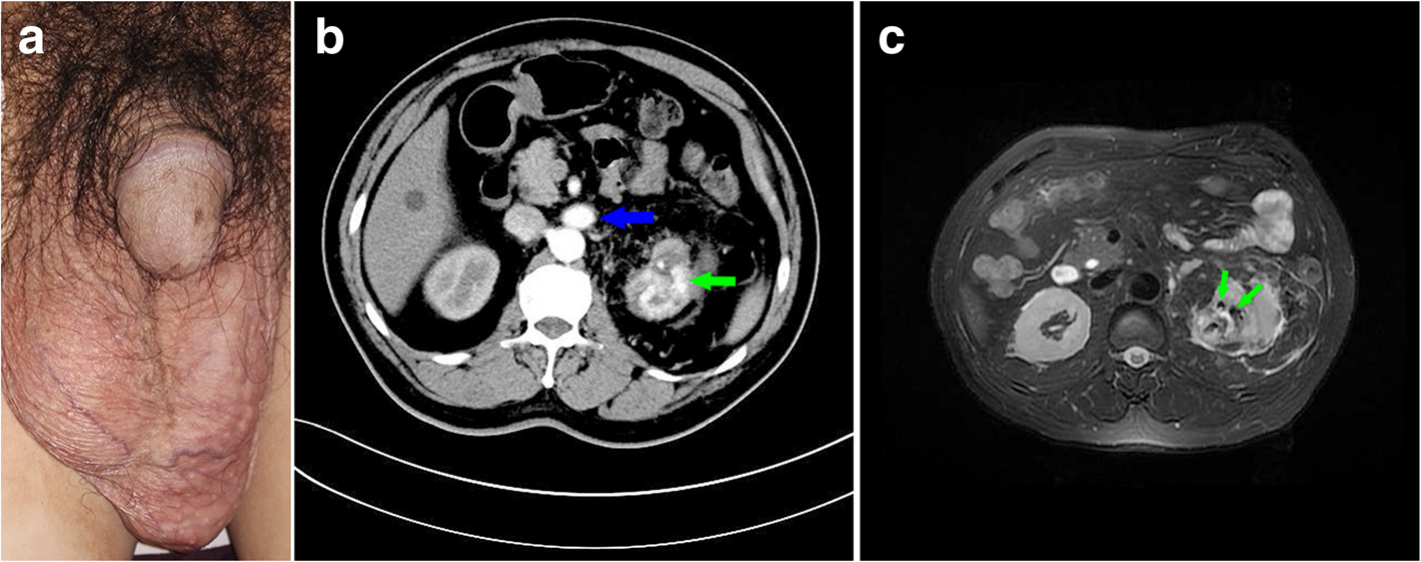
a Physical examination showed grade 3 left varicocele. b Computed tomography showed early enhanced dilated renal vein (*blue arrow*) and irregular lesion in the upper pole of left kidney, which consisted of unusual dilated enhanced vessel (*green arrow*) in renal cortical phase. c T2 magnetic resonance imaging showed abnormal early flow voids (*green arrows*) in the upper pole of left kidney
